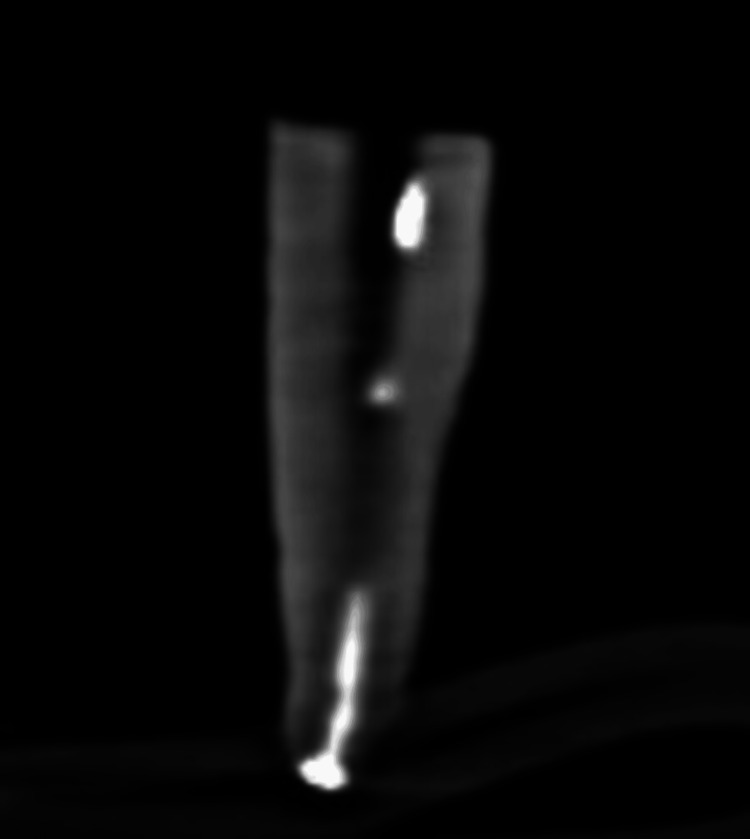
CBCT image after using the Dentsply retreatment files and obturating with the Angelus Bio-C sealer The white discontinuous shade (radio-opacity) at the center of tooth shows the remnant of the Angelus Bio-C sealer after retreatment with the Dentsply files. CBCT: cone-beam computed tomography
